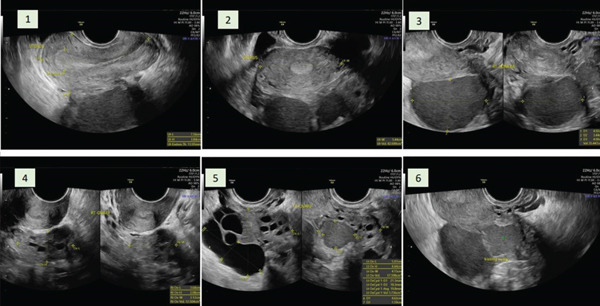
Pelvic ultrasound performed after three cycles of gonadotropin‐releasing hormone (GnRH) agonist therapy, demonstrating significant reduction in cyst size to approximately 2 cm in the right ovary and 4 cm in the left ovary. Following cyst reduction, antral follicle count could be assessed, revealing six antral follicles in the right ovary and nine in the left ovary.
